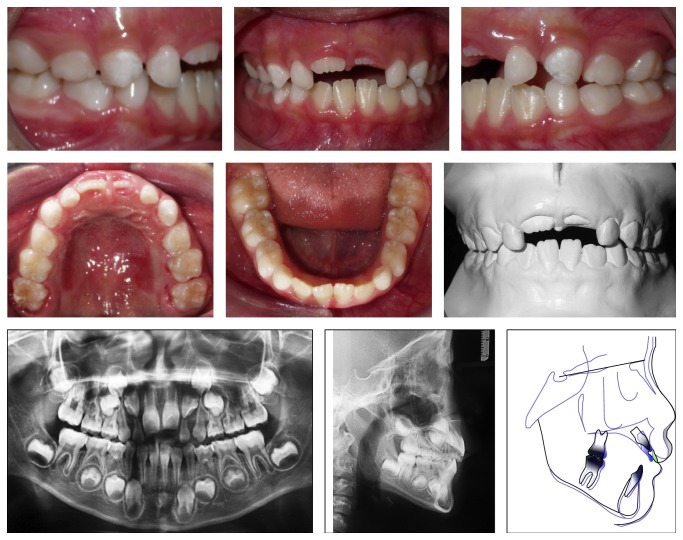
Postexpansion intraoral photographs, radiographs, and cephalometric superimposition. Black, initial; blue, final.
